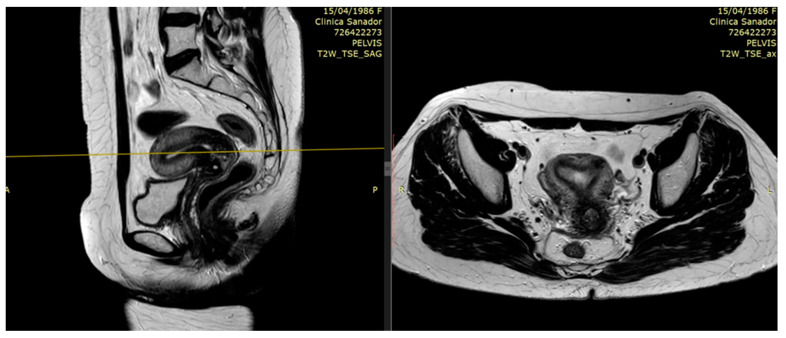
Patient 1 T2-weighted pre-brachytherapy MRI (5th week of CRT): 1.7 × 1.7 × 2.8 cm residual posterior cervix tumor and irregular tumor signal extending to left parametrium (parametrial invasion)—sagittal view and axial view.
